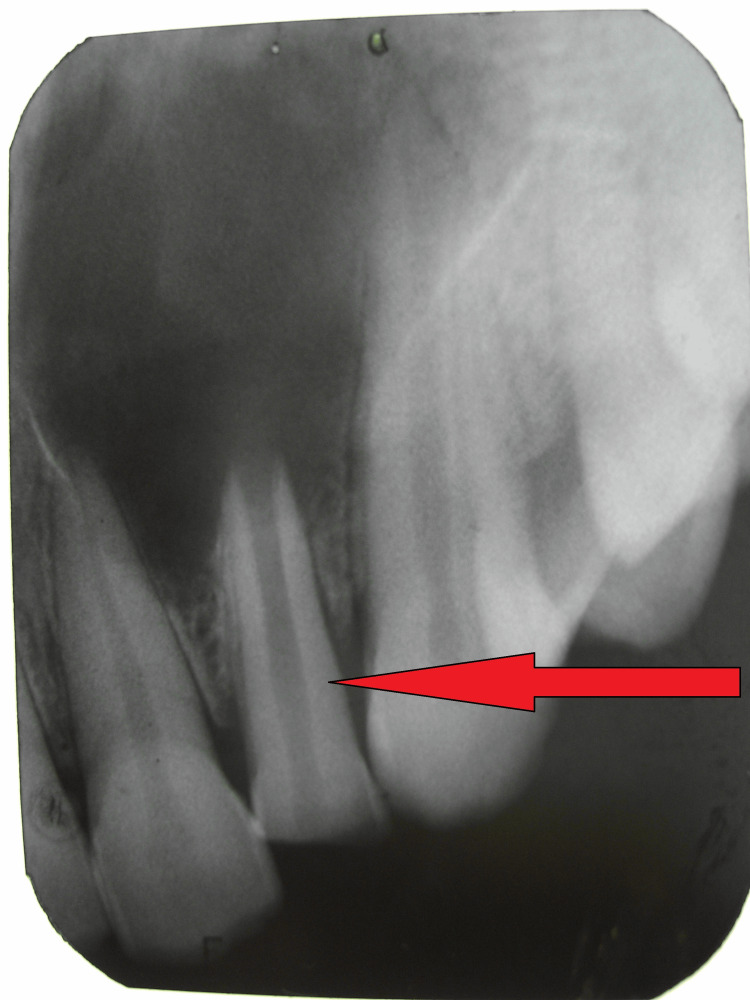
Periapical radiograph revealing ill-defined radiolucency associated with the fractured maxillary left lateral incisor (red arrow), consistent with infected periapical cyst formation.
